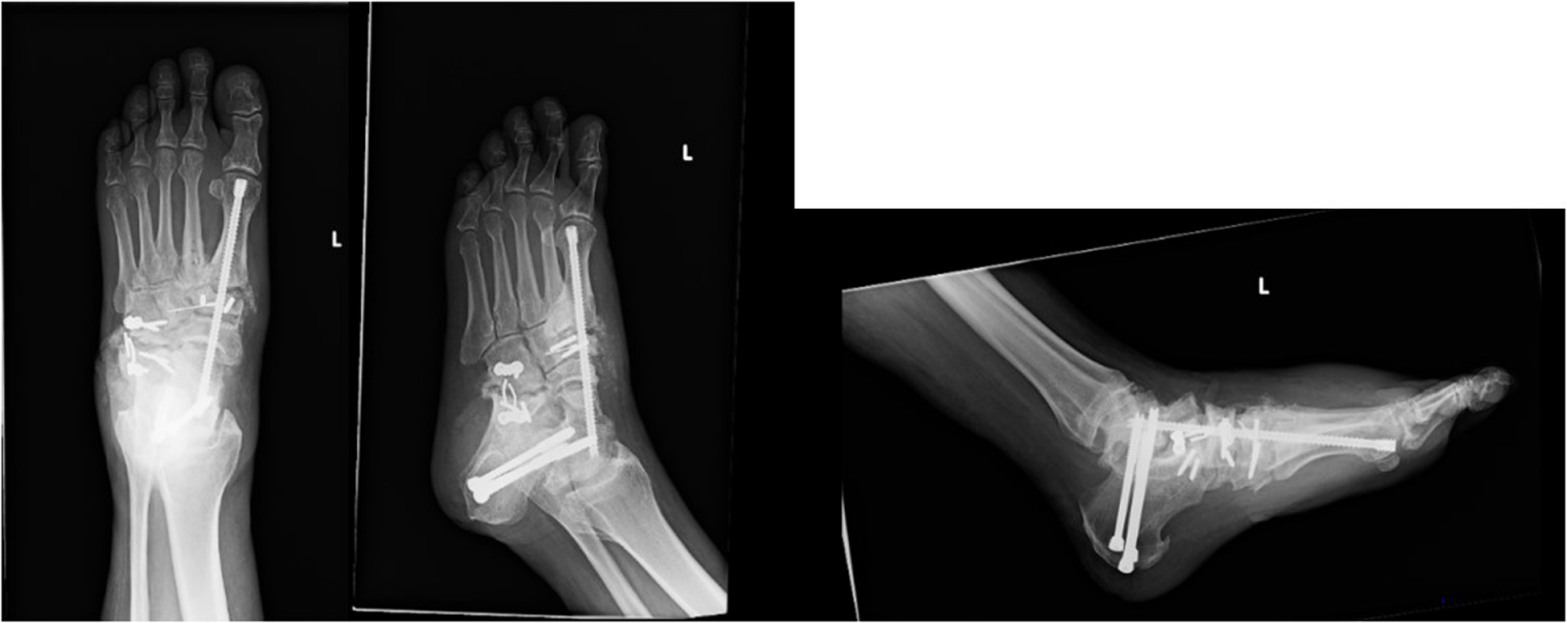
postoperative radiographs showing fusion of left foot medial column joints and the subtalar joint
